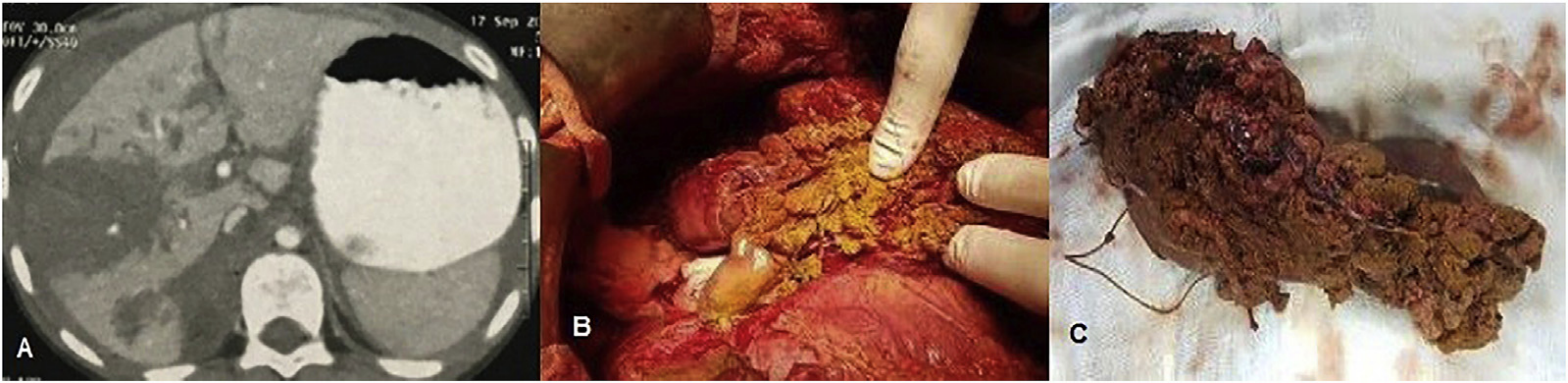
A) CT image of male patient with RTA, explored before admission and perihepatic packing was done for lacerated Rt lobe, referred to us and was explored for control of ongoing bleeding and removal of packing. B) Surgical debridement with closure of suspicious small bile ducts on cut surface with proline 5/0 and drain inserted. C) The resected part of Rt lobe.
